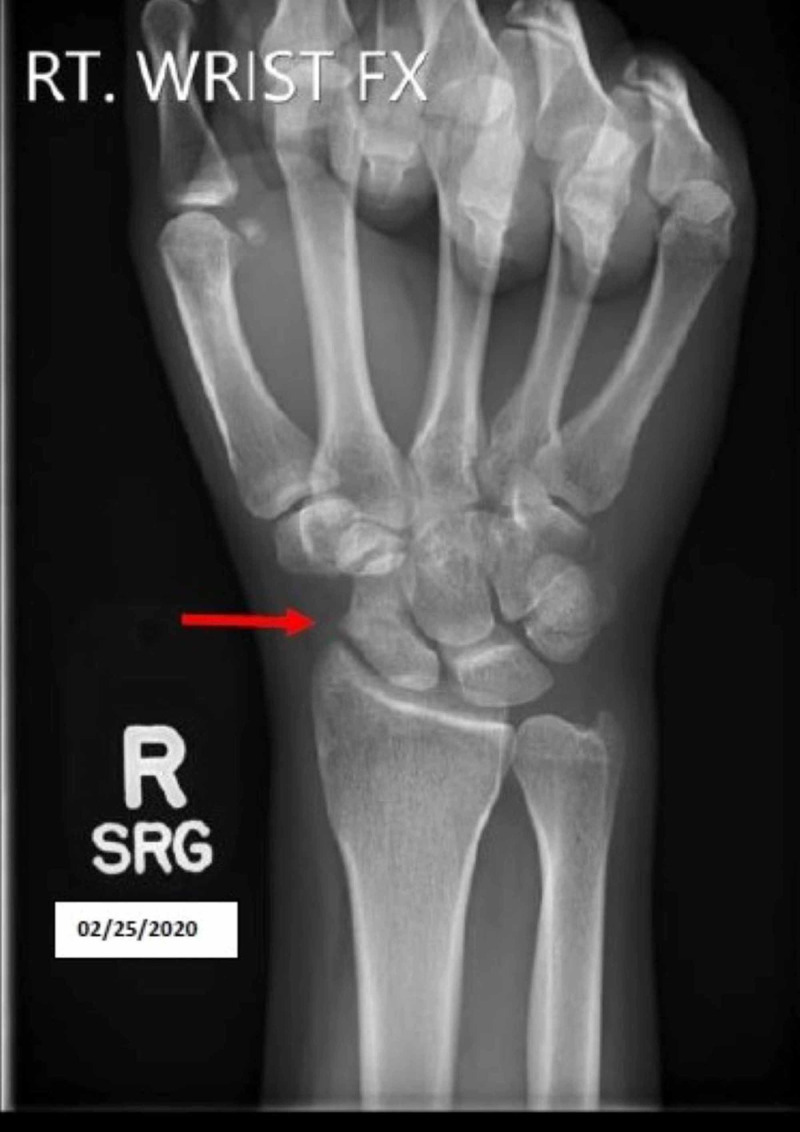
X-ray revealed increased healing of scaphoid waist fracture with mild displacement, overall good alignment, and continued callus formation with still incomplete healing (Red arrow)
